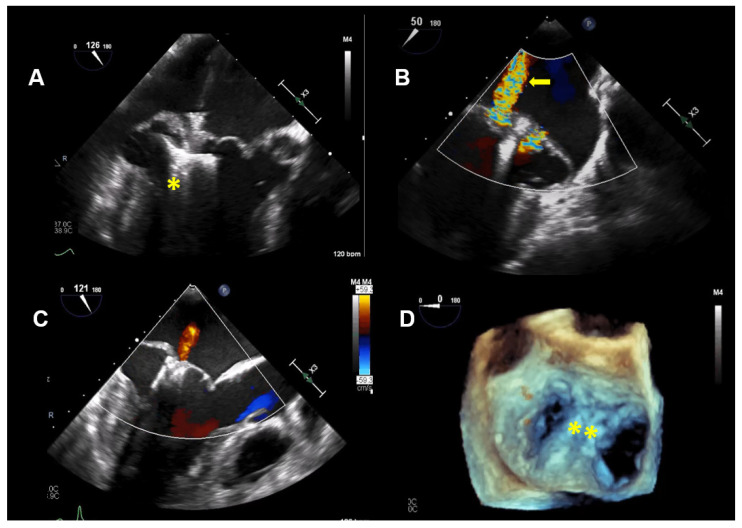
Case 2: Procedural transesophageal echocardiographic imaging during MitraClip XTR (Abbott Vascular, North Chicago, Illinois, USA) implantation. (A) The first MitraClip XTR (yellow asterisk) is positioned below the mitral leaflets with opened Clip arms. (B) Residual mitral regurgitation (MR) after implantation of the first Clip is marked with a yellow arrow. (C) After positioning of the second Clip, only mild MR is present. (D) The 2 MitraClip XTR devices (yellow asterisk), which create a double orifice, are seen in a 3D enfaced view.
